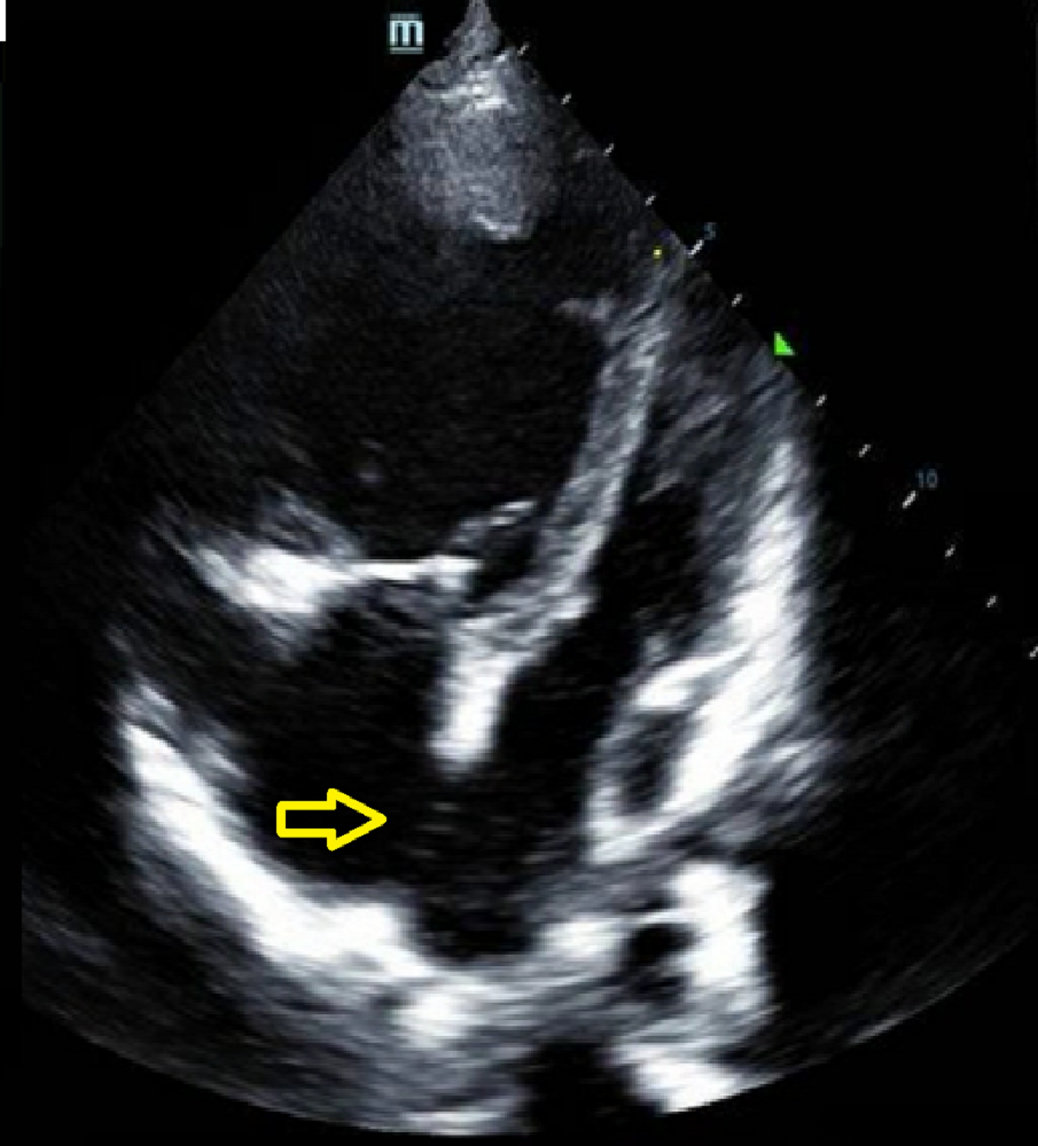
Apical four-chamber view transthoracic echocardiogram showing atrial secundum defect Arrow: atrial secundum defect
